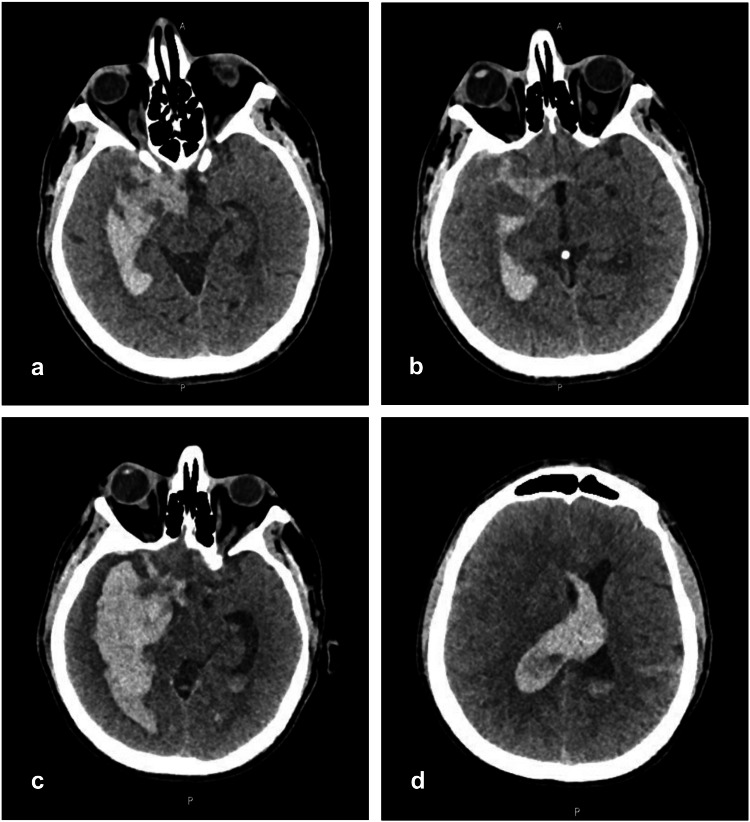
Noncontrast head computed tomography (CT) scan (a-b) Scan obtained on admission demonstrating subarachnoid hemorrhage in the suprasellar cistern with intraventricular extension; (c-d) Significant increase in the size of intraventricular hemorrhage with a midline shift of 10 mm
